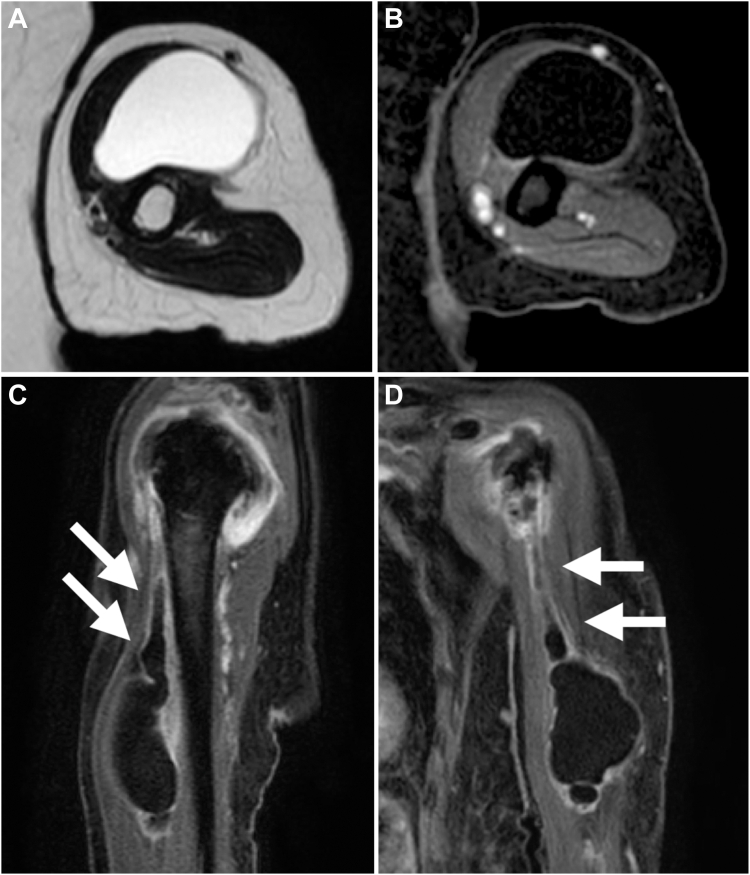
Preoperative magnetic resonance images of the left upper arm. (A) Axial images showed a homogenous left upper arm mass, measuring 6 × 4 cm, with a hyperintense signal on T2-weighted imaging. (B) The mass exhibited a hypointense signal on fat-suppressed T1-weighted imaging and was not enhanced. (C) Sagittal images showed a proximal extension of the mass. (D) Coronal images revealed that the proximal end of the mass was connected to the bicipital groove along with the long head tendon of the biceps brachii muscle.
